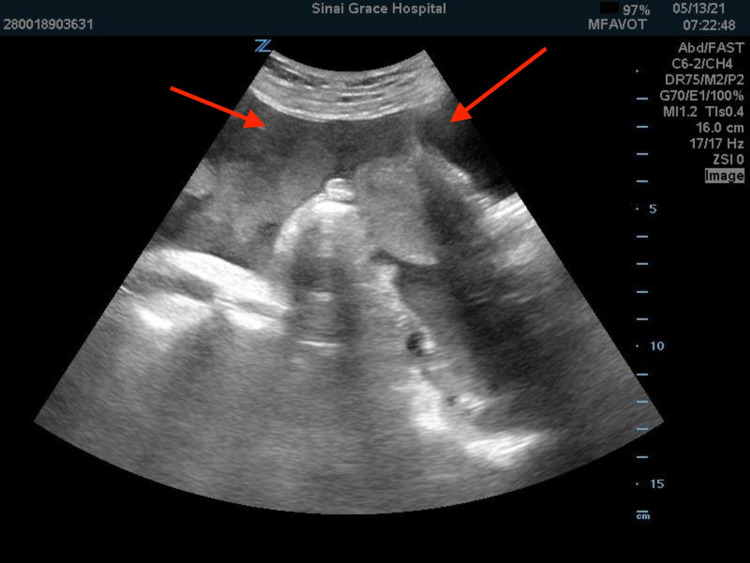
Bedside FAST exam demonstrated complex echogenic fluid in the abdomen, concerning for hemoperitoneum (arrows). FAST, focused assessment with sonography in trauma.
